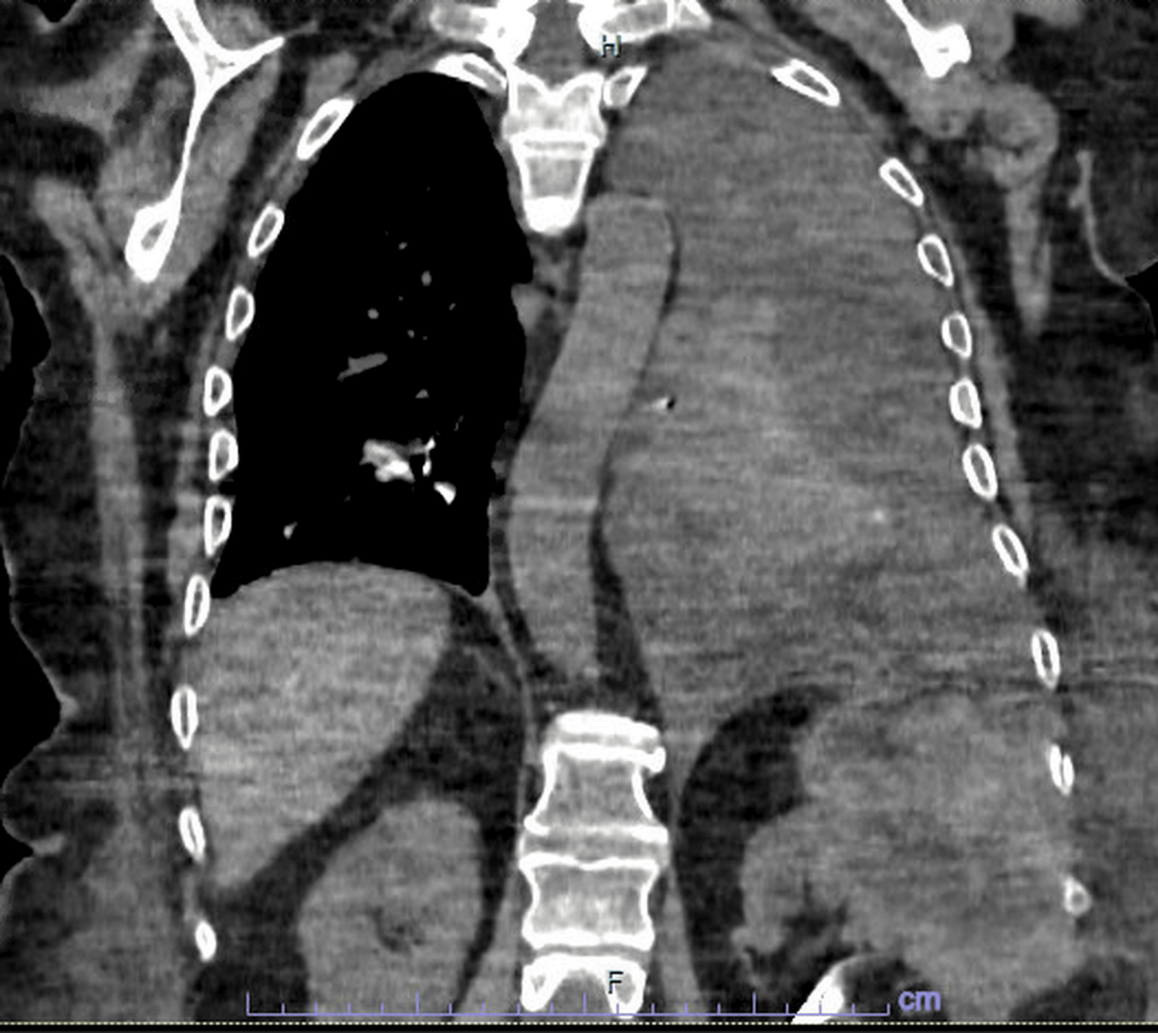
Thoraco-abdominal CT scan revealing a superinfected and fistulized left renal mass to the pleura, resulting in a large pyothorax
